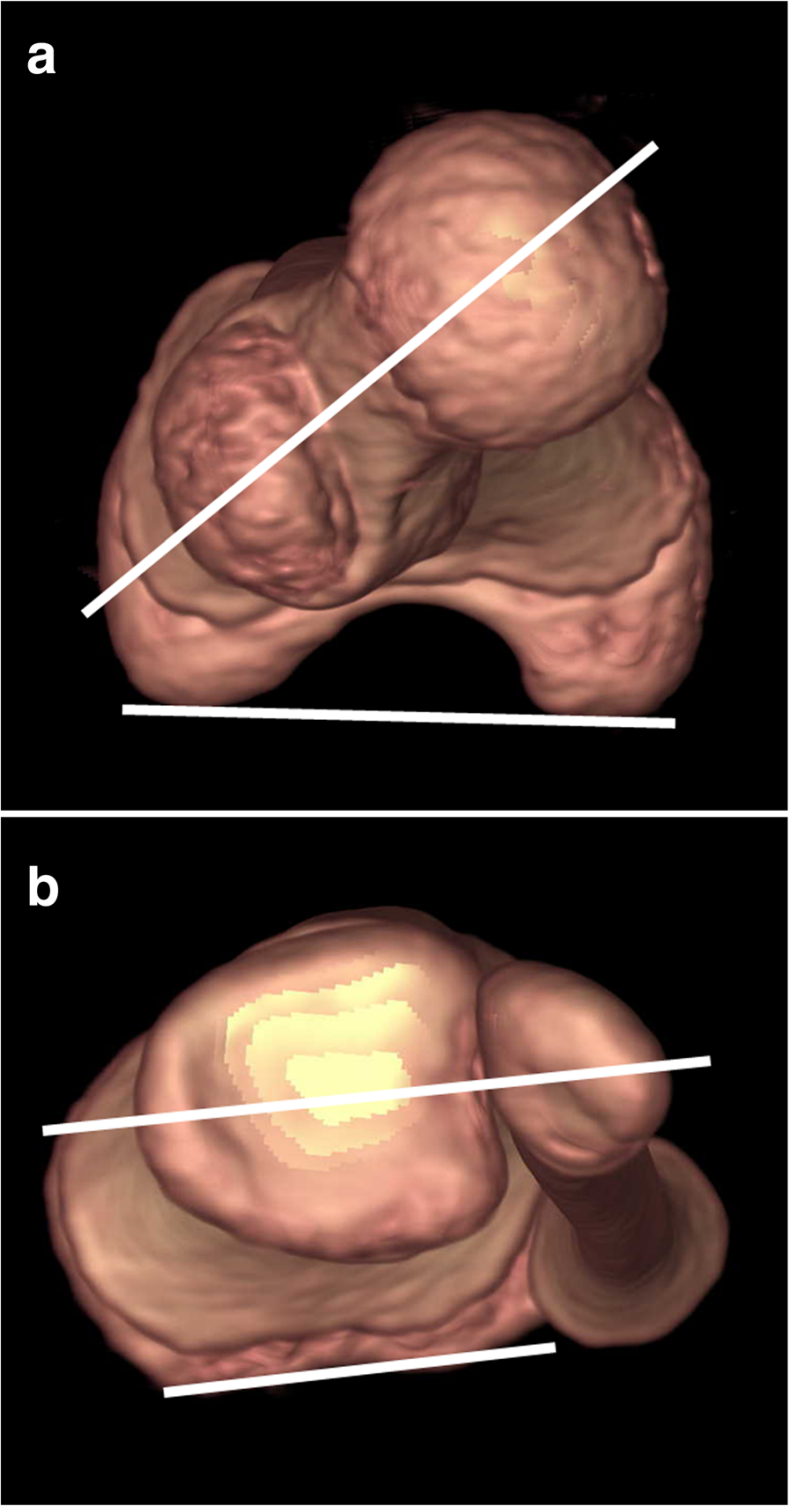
Axial three-dimensional computed tomography scans (A) Femoral anteversion is the angle between the line connecting the centers of the femoral head and greater trochanter and another line connecting the posterior margins of the medial and lateral femoral condyles. (B) Tibial torsion is the angle between the line connecting the posterior margins of the medial and lateral tibial condyles and another line connecting the midpoints of the medial malleolus and syndesmotic articular surface of the lateral malleolus
